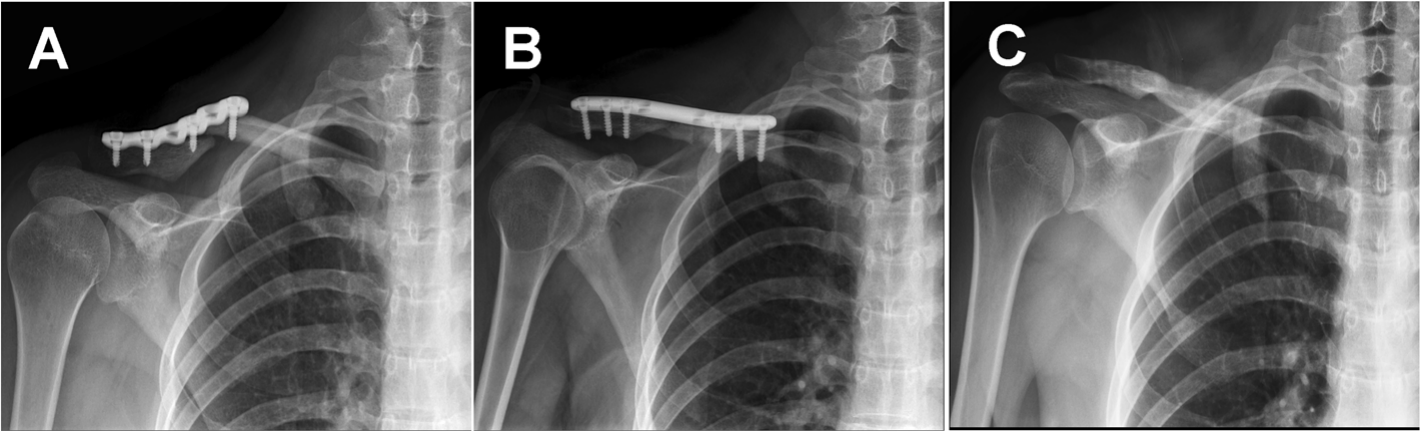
a Pre-operative radiograph of a clavicle fracture non-union (atrophic) in a 31-year-old female. b Immediate post-operative radiograph showing fixation with three screws on either side of the fracture. c One year after surgery, the internal fixation devices were removed. The final follow-up radiograph showing fracture union
